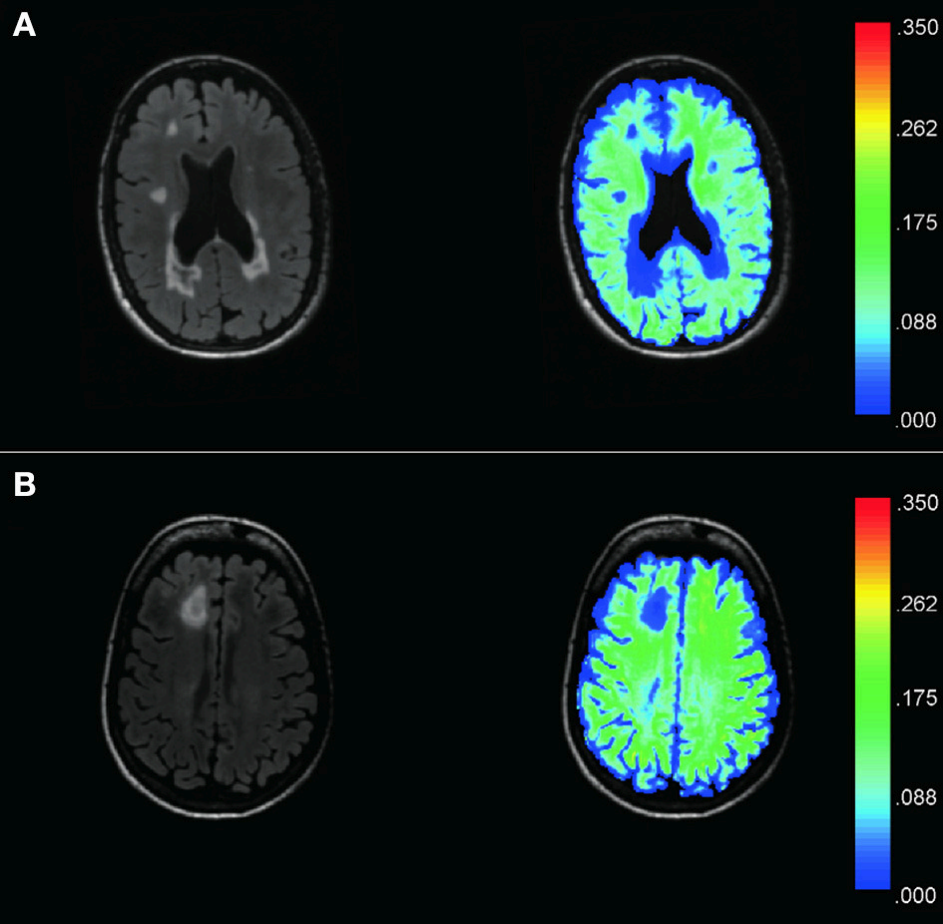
Examples of T2 FLAIR images (left) from two MS patients (A,B) and corresponding MWF maps (right) demonstrate good spatial resolution and sensitivity to visually T2 hyperintense lesions. The color bar represents the range of MWF values. Hyperintense lesions on T2 Flair images are found to have low MWF values as compared to the surrounding NAWM. MWF also appears sensitive to dirty WM, as shown by the low MWF displayed in dark/light blue within the central white matter of (B).
